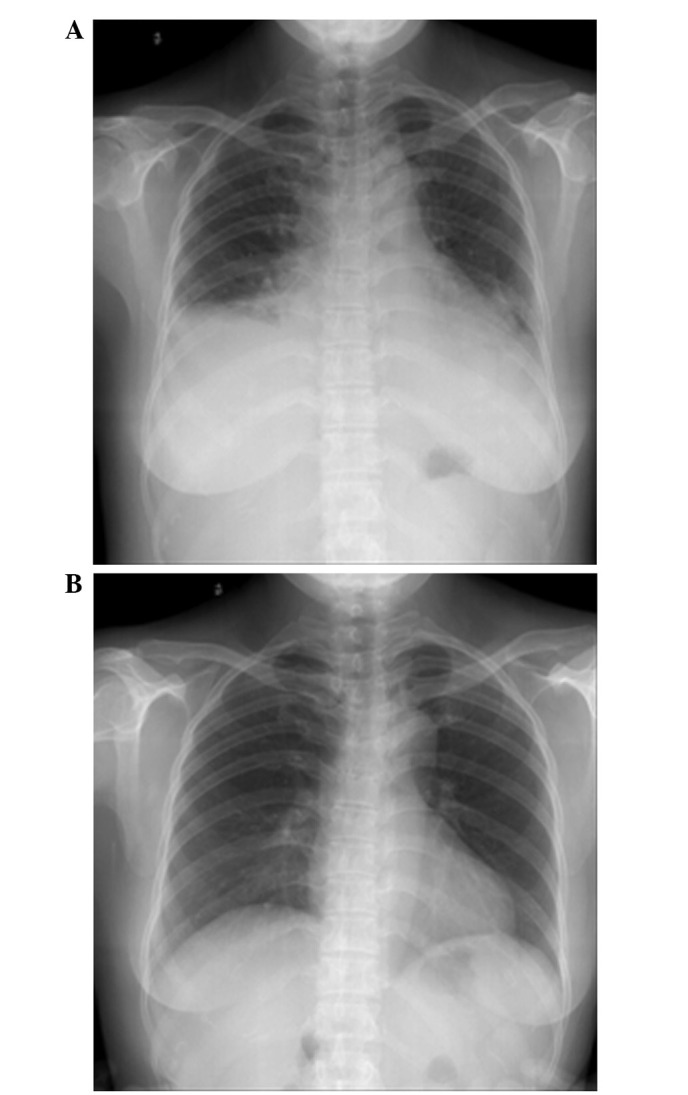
(A) Post-operative chest radiograph showing a small amount of bilateral pleural effusion, infection in the bilateral lower lungs and ardiac enlargement. (B) Pre-operative chest radiograph showing no cardiopulmonary abnormalities.
